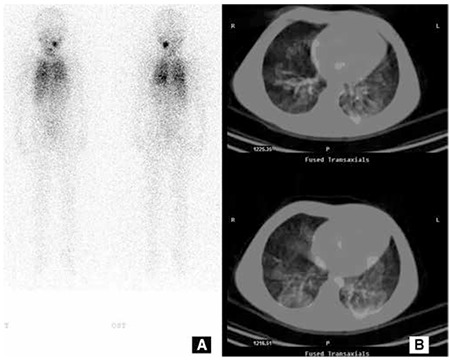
I-131 scintigraphy of a 6 year-old girl who received a total of 250 mCi I-131 RAI therapy showed radioactivity stasis in the left submandibular gland, and bilateral lung metastases on wholebody imaging (A). The SPECT/CT fusion imaging revealed that the lung metastases were not miliary. A 150 mCi RAI therapy was planned
